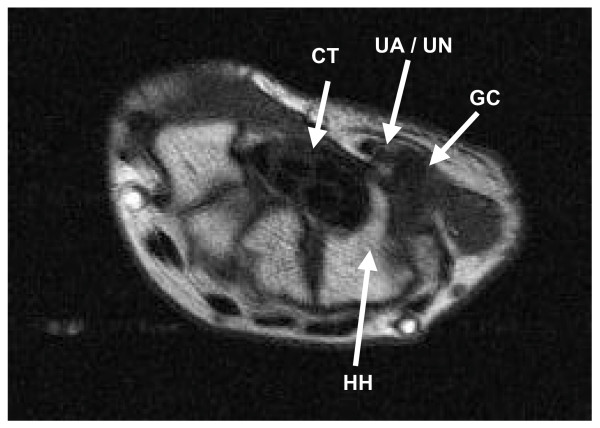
MRI scan of the right wrist showing a ganglion cyst (GC) in the region of the ulnar artery and nerve (UA/UN) medial to the right carpal tunnel (CT) and hook of hamate (HH).
